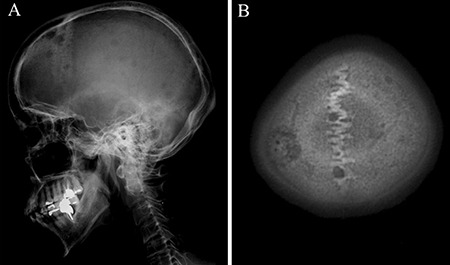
A, B. Lateral skull radiograph showing punched-out lesions (A), and computed tomography of the head showing multiple osteolytic lesions (B).
